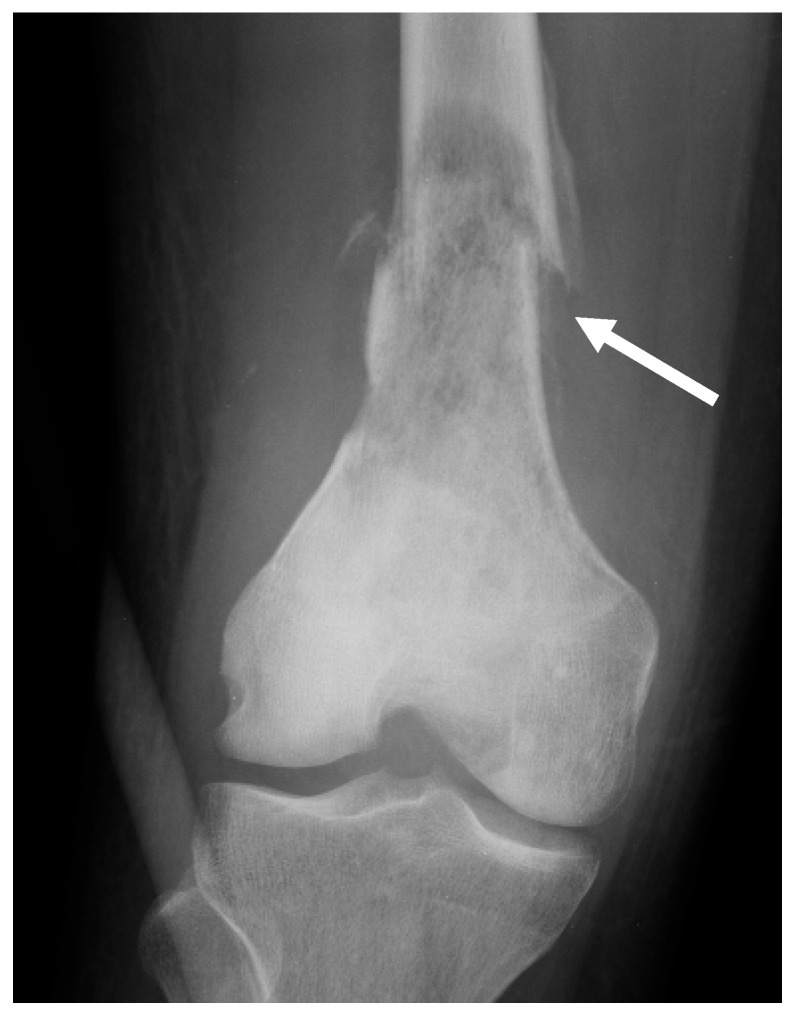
Pathological fracture on conventional radiography (AP view) of a 22-year-old male diagnosed with conventional (fibroblastic) osteosarcoma taken during neoadjuvant chemotherapy (arrow).
